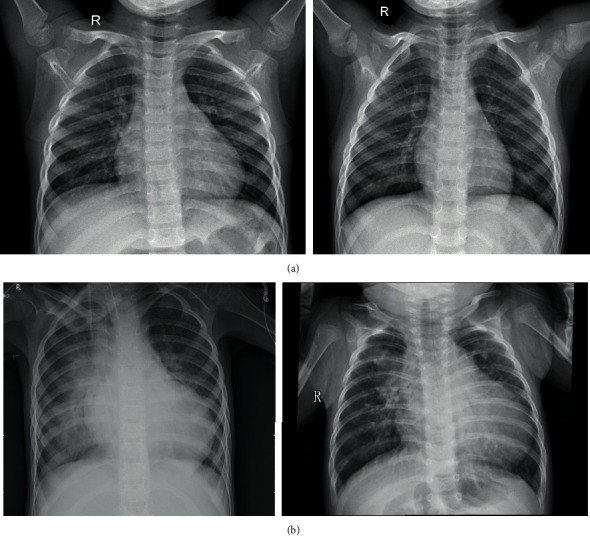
Pneumonia X-ray image: (a) image of pneumonia sufferers; (b) nonpneumonia images.
